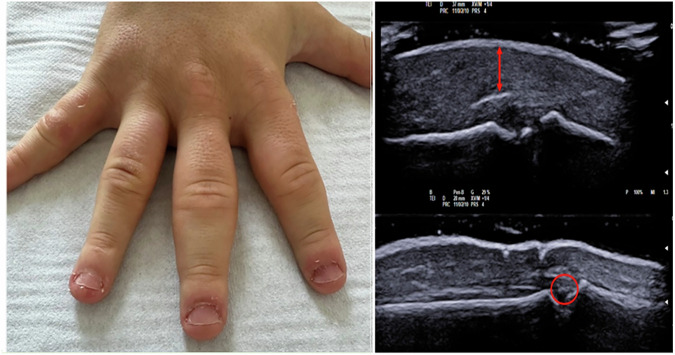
Dactylitis in pachydermodactyly. Left: Chronic, painless swelling of the third finger in an adolescent male. Right: Ultrasound images showing subcutaneous soft tissue thickening (arrow) and, on palmar longitudinal scan, absence of joint capsule distension or extensor tendon inflammation (circle).
